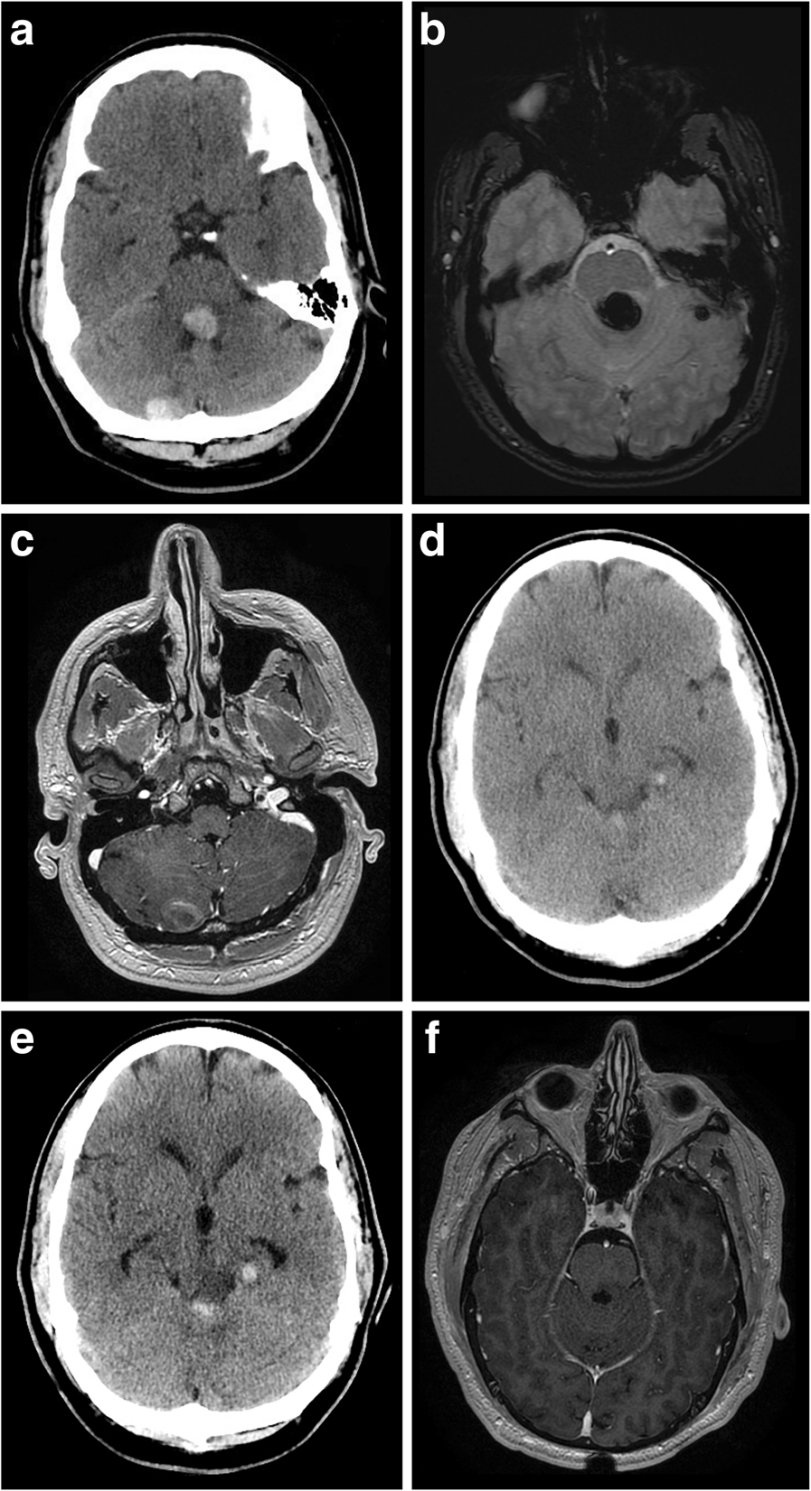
Cranial imaging of multiple cerebral cavernous malformations. a Computed tomography scan indicating two areas suspicious for acute hemorrhage: one within the fourth ventricle and the other adjacent to the calvarium in the patient’s right cerebellum. b Gradient echo magnetic resonance imaging showing lesion in left lateral cerebellar hemisphere. c Magnetic resonance imaging with contrast showing heterogeneous enhancement in the right cerebellar mass. d Computed tomography scan demonstrating increased hemorrhage and size within the ventricular lesion and the new hemorrhagic hyperdensity within the left medial temporal location. e Computed tomography scan displaying further hemorrhagic enlargement of the intraventricular and temporal lesions with development of hydrocephalus. f Postoperative magnetic resonance imaging showing complete resection of fourth ventricular and right cerebellar masses
